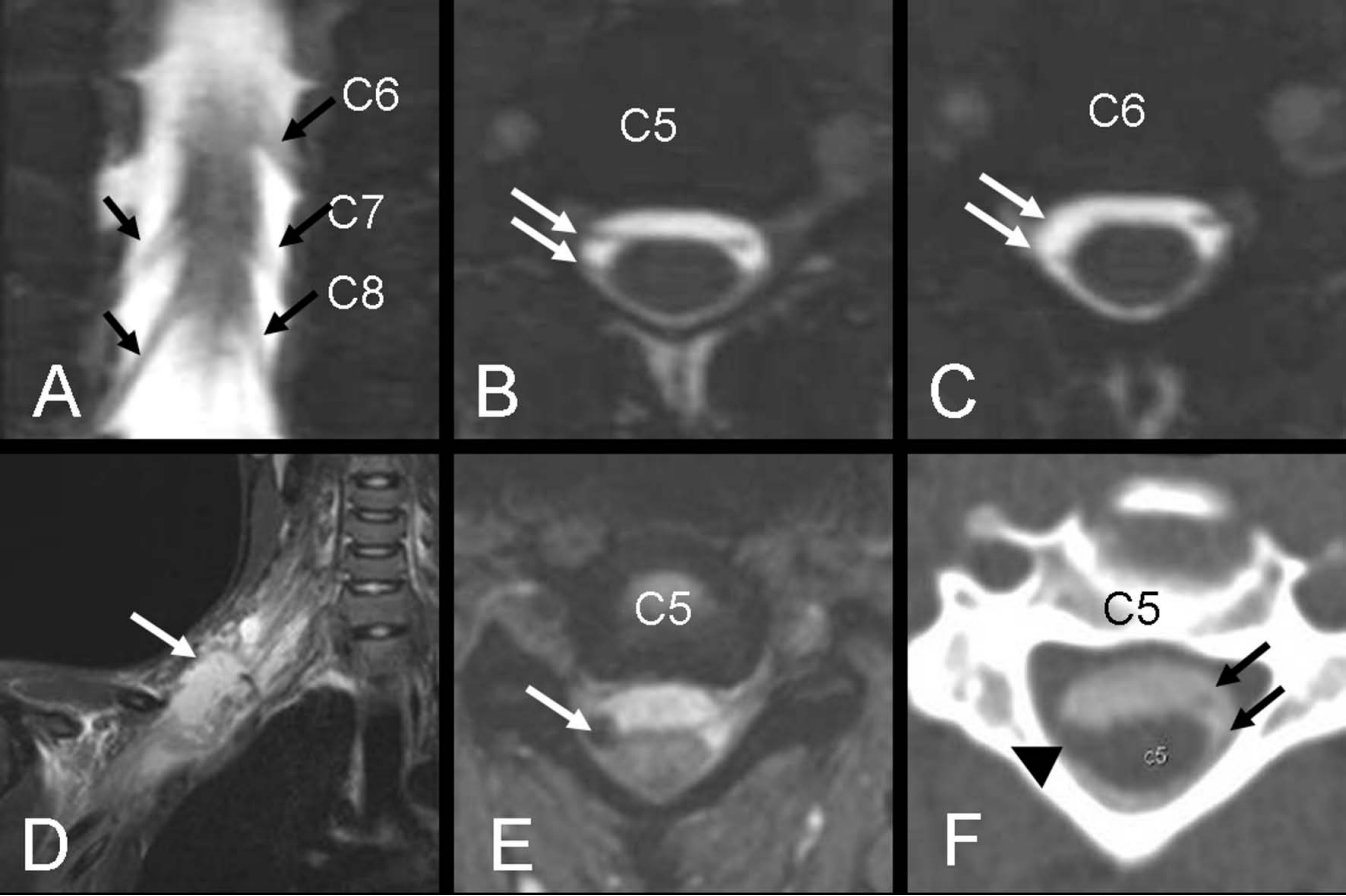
(A-C) MRI 3D CISS of patient No 1. The Coronal image (A) shows avulsion of C6 root on the right side. The intact roots are marked with arrows. Axial images (B-C) show normal C5 roots (arrows, B) and avulsion of C6 roots on the right side (arrow, C). However, exploration revealed avulsion of both C5 and C6 on the right side (false negative MRI-finding at C5). (D-F) Images of patient No 2. The coronal STIR (D) shows edema around the supra- and infraclavicular plexus. (E) Axial turbo flash image shows extremely low signal at the C5 root exit indicating bleeding. (F) Axial CT-M shows hematoma at the site of dorsal root exit (arrow head) and absence of ventral root. Black arrows show the normal C5 roots on the left side. Similar findings were revealed at the level of C6 and C7. MRI findings were concordant with the intraoperative findings.
